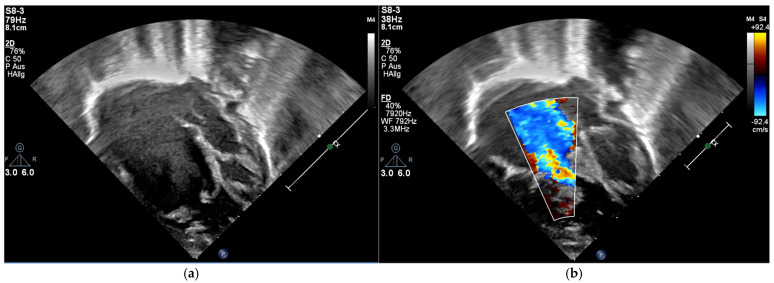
Echocardiography, four-chamber view; (a) the apical displacement of the tricuspid valve; (b) color-flow Doppler showing severe tricuspid regurgitation; immediately postpartum.
